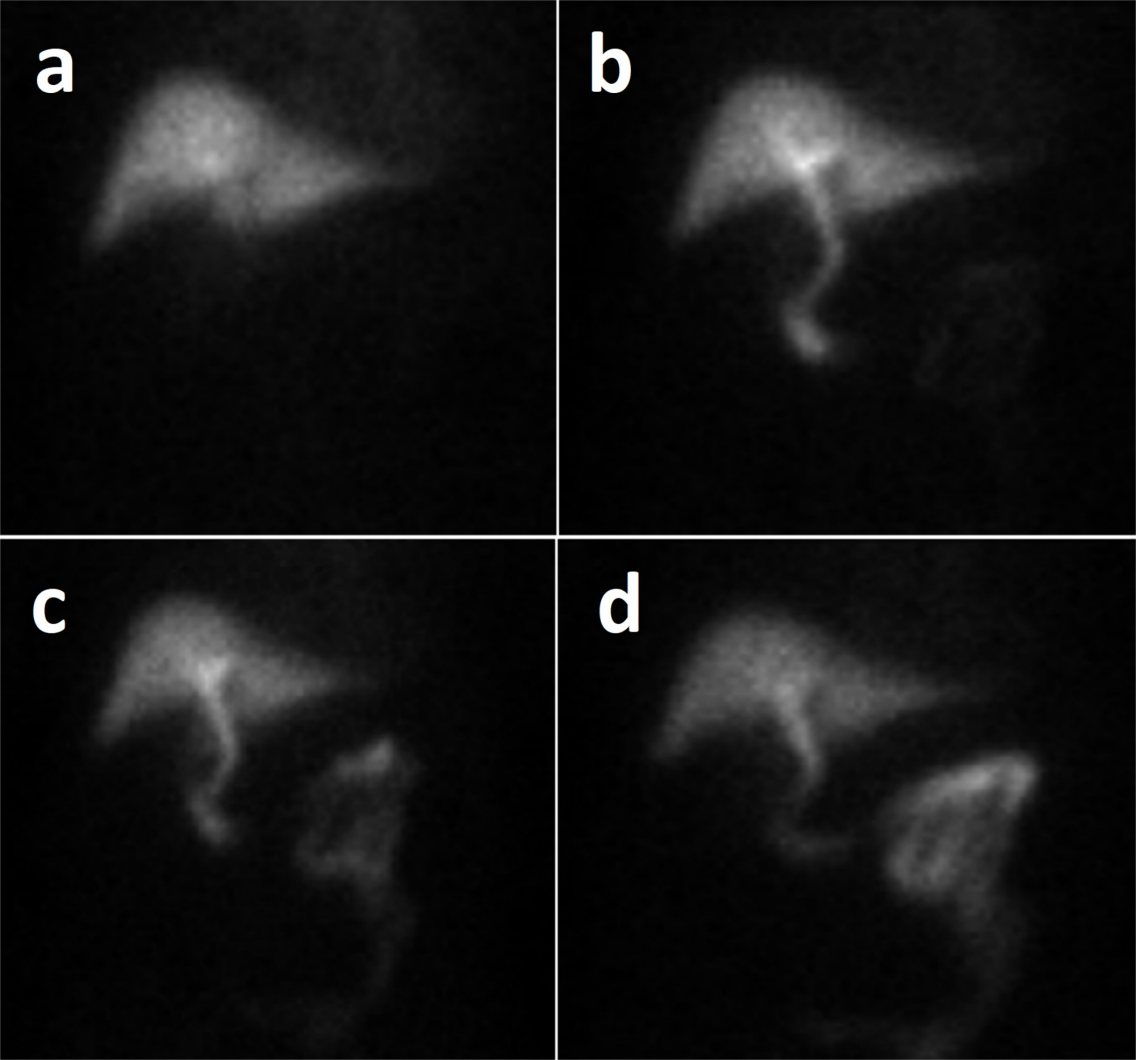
Dynamic hepatobiliary scintigraphy (HIDA) scan images of our patient taken at 5 minutes (a), 20 minutes (b), 40 minutes (c), and 60 minutes (d) intervals. The gall bladder could not be visualized, even after 60 minutes (d).
